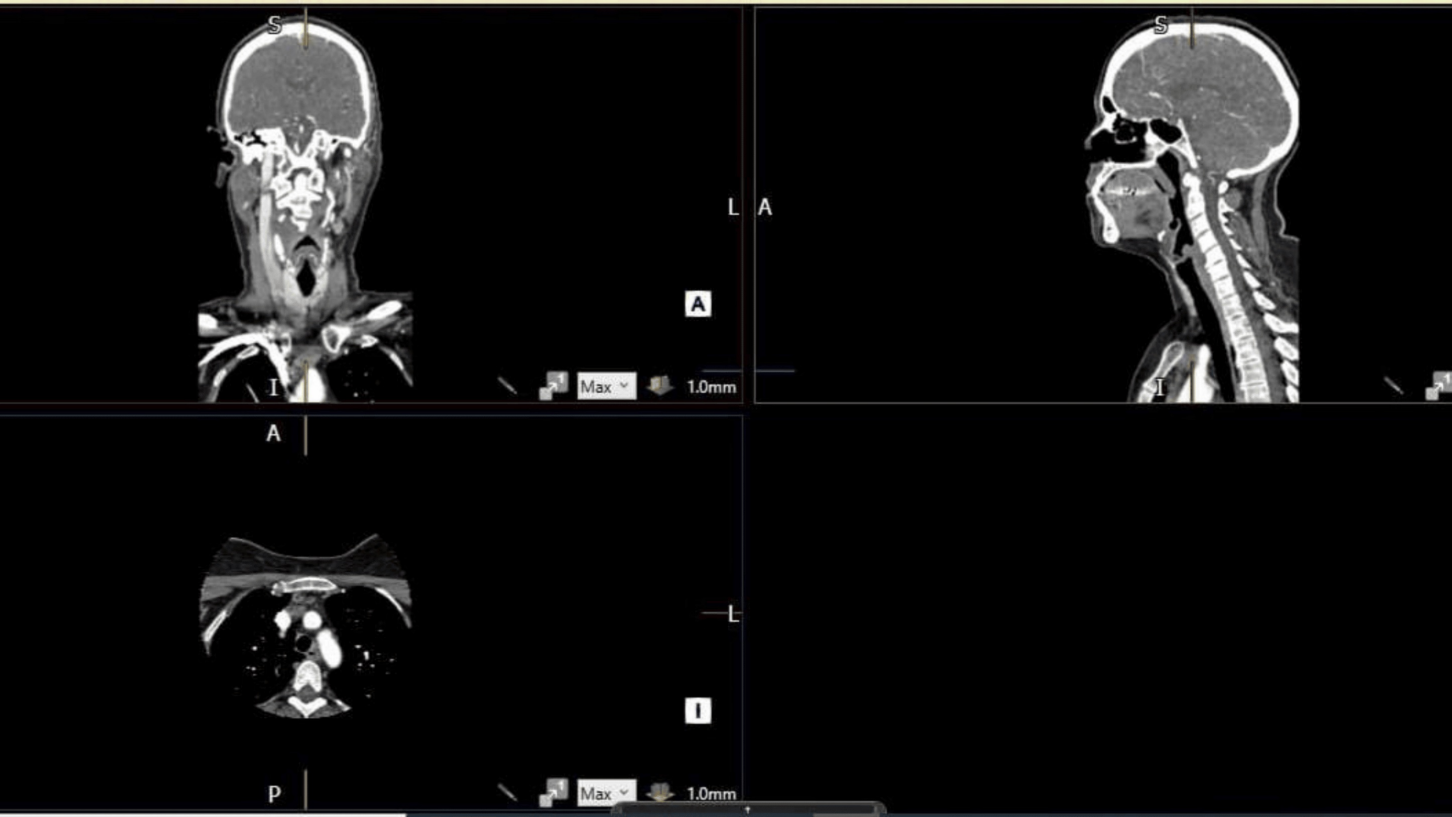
Computed tomography of the aortic arch and carotid angiogram indicate no significant stenosis 3D imaging
